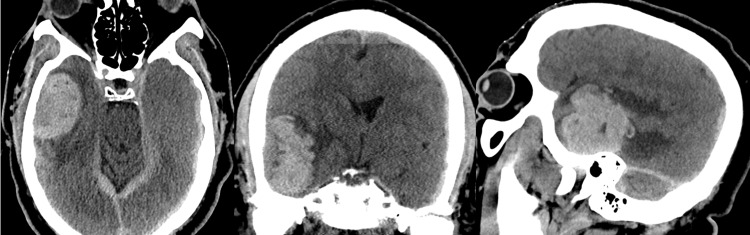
Multiplanar non-contrast head CT in a sexagenarian male presenting with progressive confusion and loss of consciousness. CT demonstrating a large, dural-based hemorrhage at the right temporal convexity with bilateral tentorial and hemispheric subdural hematomas and parafalcine subdural hematoma. Regional mass effect with right to left subfalcine shift is also evident.
